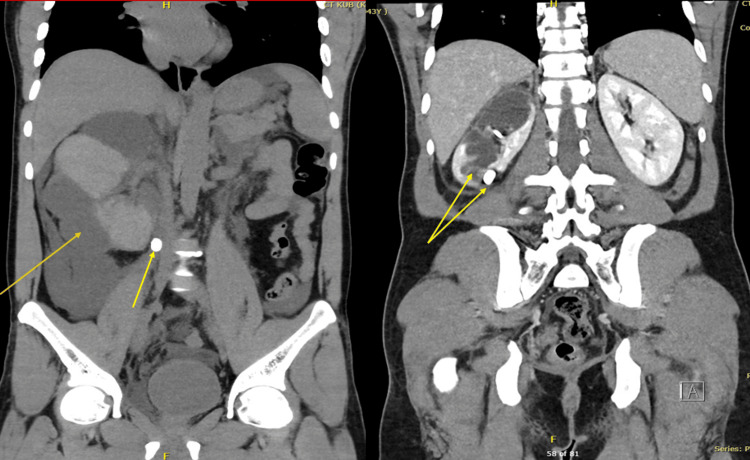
Two coronal-view CT scans show the abdomen before and after a medical procedure. The first scan reveals a large fluid collection (urinoma/hematoma), indicated by the left yellow arrow, and a proximal kidney stone on the right side (central yellow arrow). The second scan, a follow-up, demonstrates that the fluid collection has significantly reduced and the stone has moved into the lower part of the kidney (lower yellow arrow).
